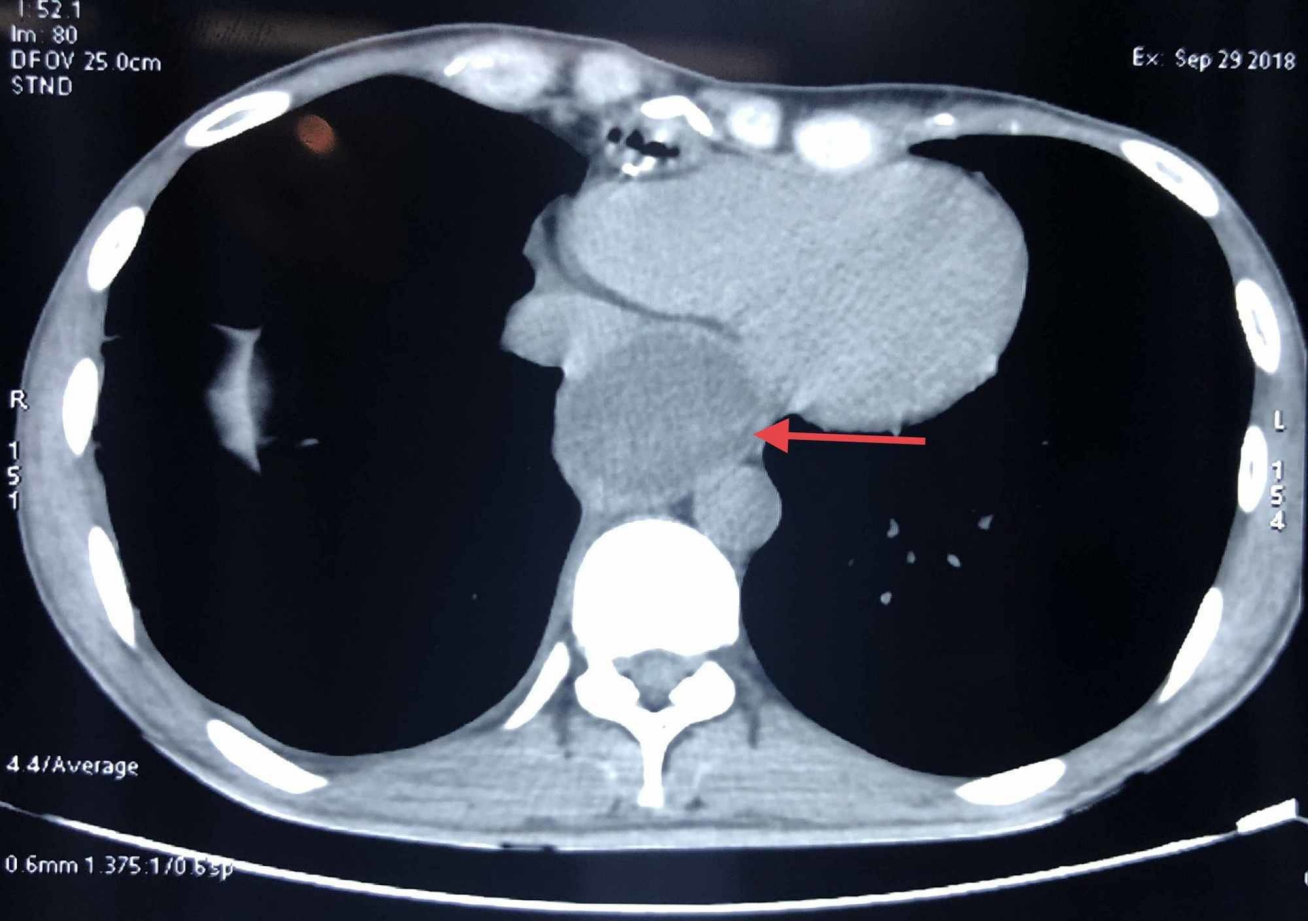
CT thorax showing the mucocele of the remnant esophagus
